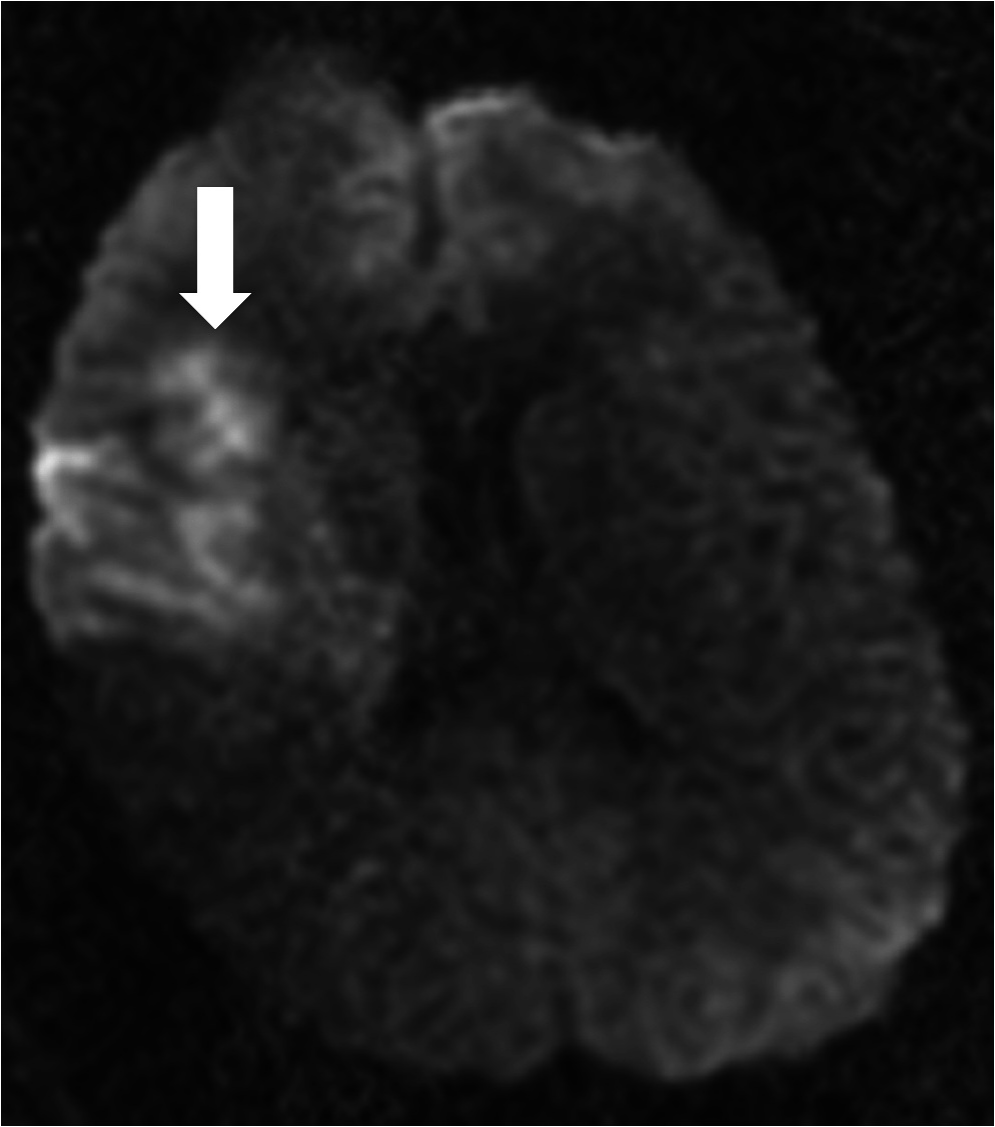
Diffusion-weighted image from a brain magnetic resonance image on the day of presentation reveals an acute infarct in the right middle cerebral artery territory.
